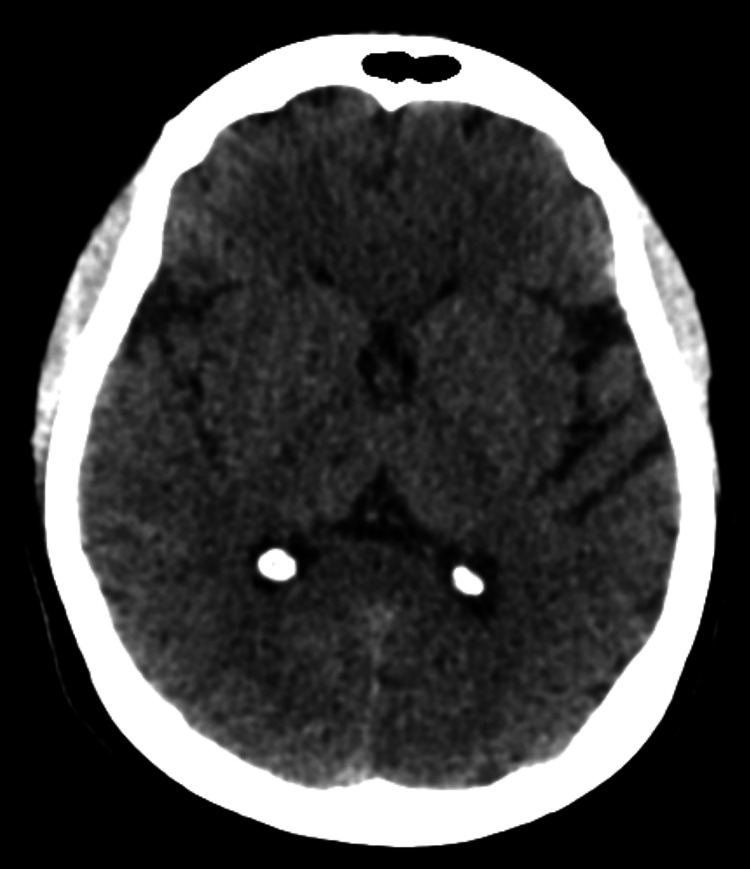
CT head without contrast does not show any thalamic infarct.
